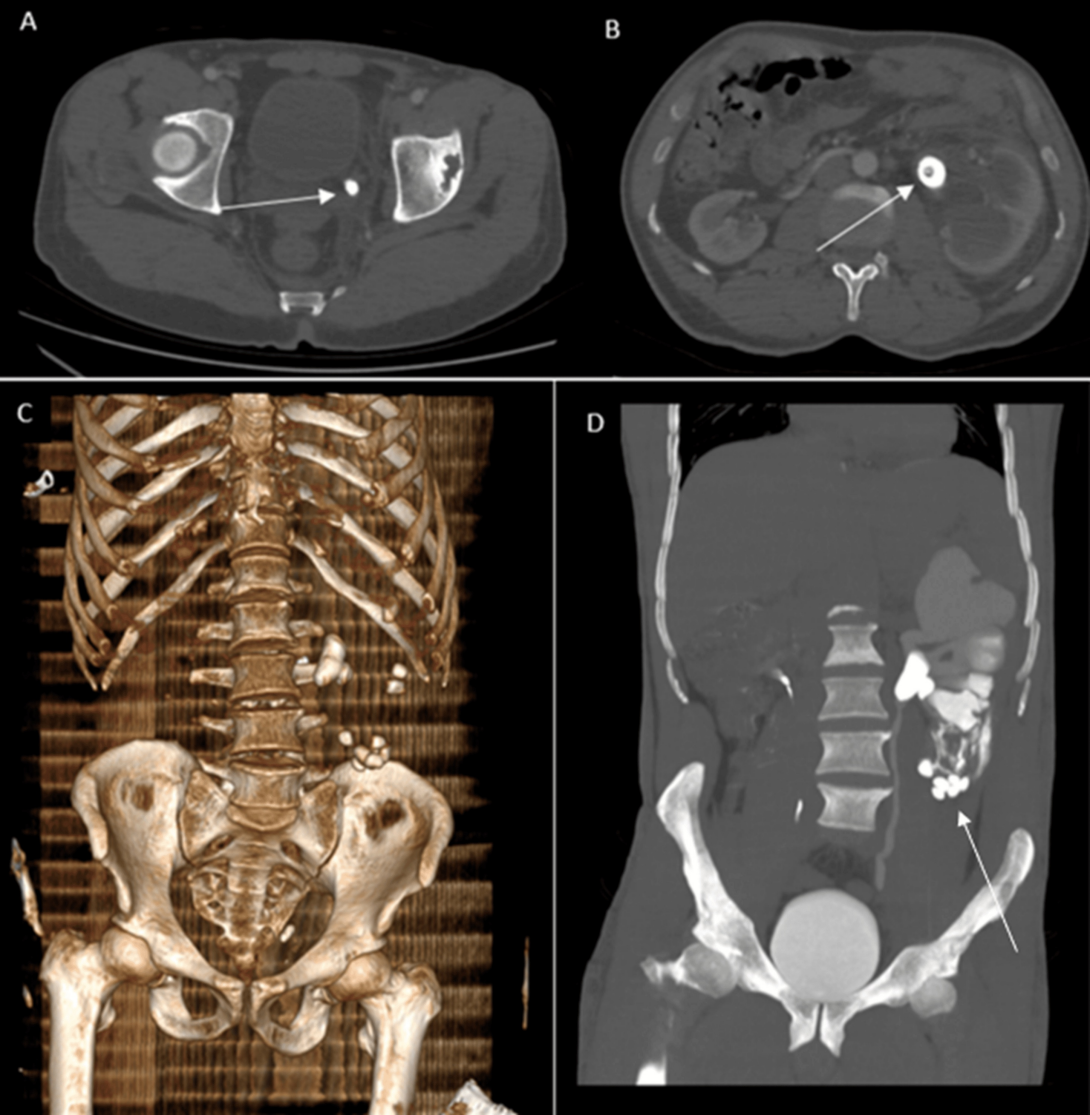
Imaging studies (A) Axial CT image in bone window during the arterial phase showing a distal ureteral stone; (B) Axial CT image in bone window during the arterial phase, demonstrating a large staghorn calculus within the renal pelvis; (C) Three-dimensional volume-rendered reconstruction depicting multiple renal and ureteral calculi; (D) Coronal maximum intensity projection (MIP) reconstruction in the delayed phase showing multiple calculi and marked left hydronephrosis. Some stones appear extrarenal, displaced beyond the renal capsule.
